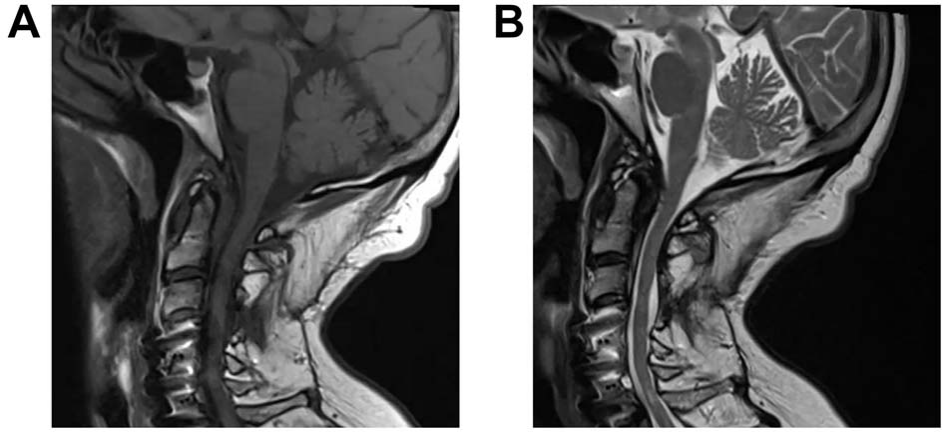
Repeat MRI 4 months post-minimal access far-lateral transcondylar approach for resection of the C1-2 degenerative cyst; sagittal T1-weighted (A) and T2-weighted (B) sequences demonstrate gross total resection of the cyst with complete resolution of the medullary syrinx.
